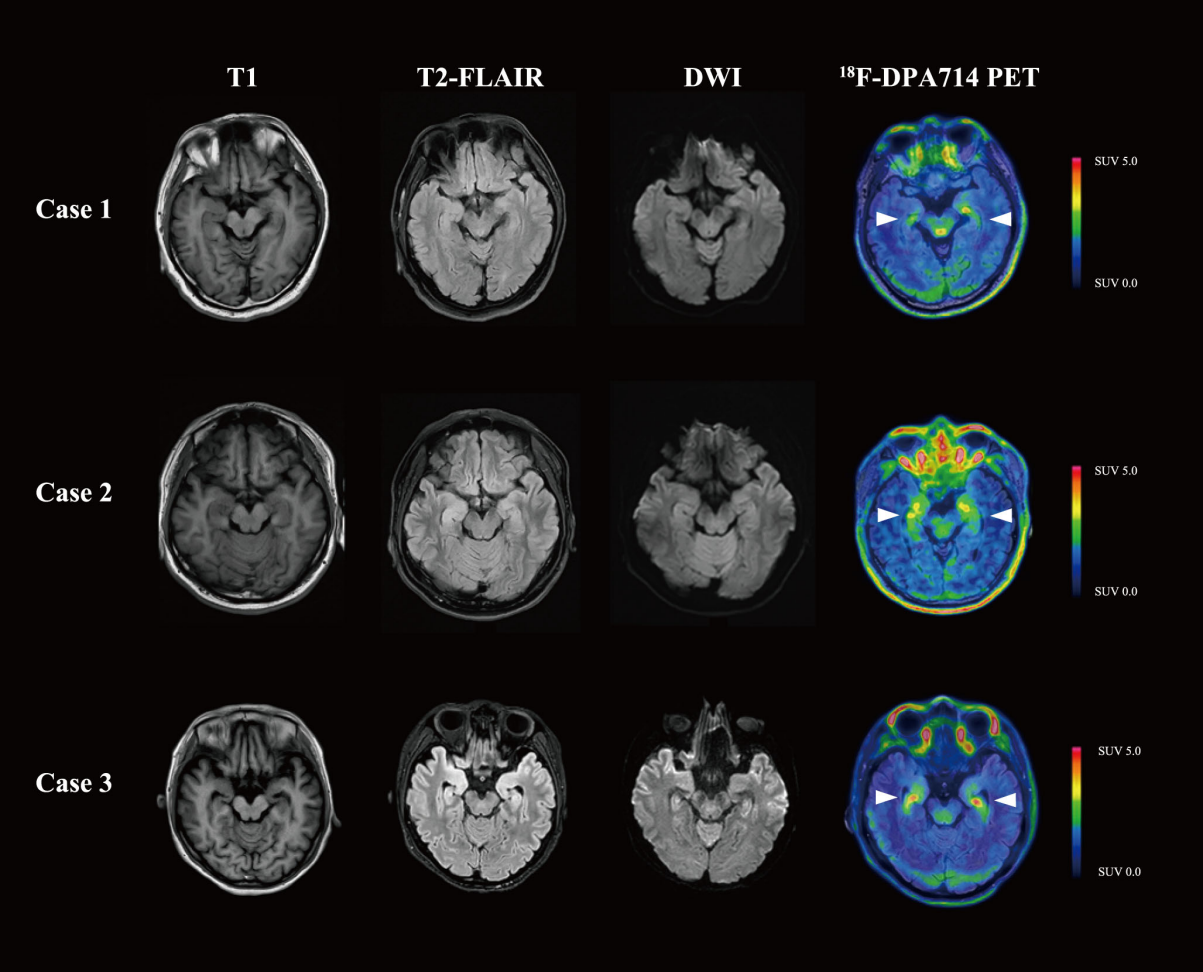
Brain MRI and ^18^F-DPA714 PET/MRI images of the three patients. Cases 1 and 2 presented with normal MRI, while Case 3 was found to have T2-FLAIR and DWI hyperintensity in the bilateral medial temporal lobes. In ^18^F-DPA714 PET/MRI, all three patients had elevated uptake in the bilateral medial temporal lobes. T1, T1-weighted imaging; T2-FLAIR, T2-weighted fluid attenuated inversion recovery; DWI, diffusion weighted imaging.
